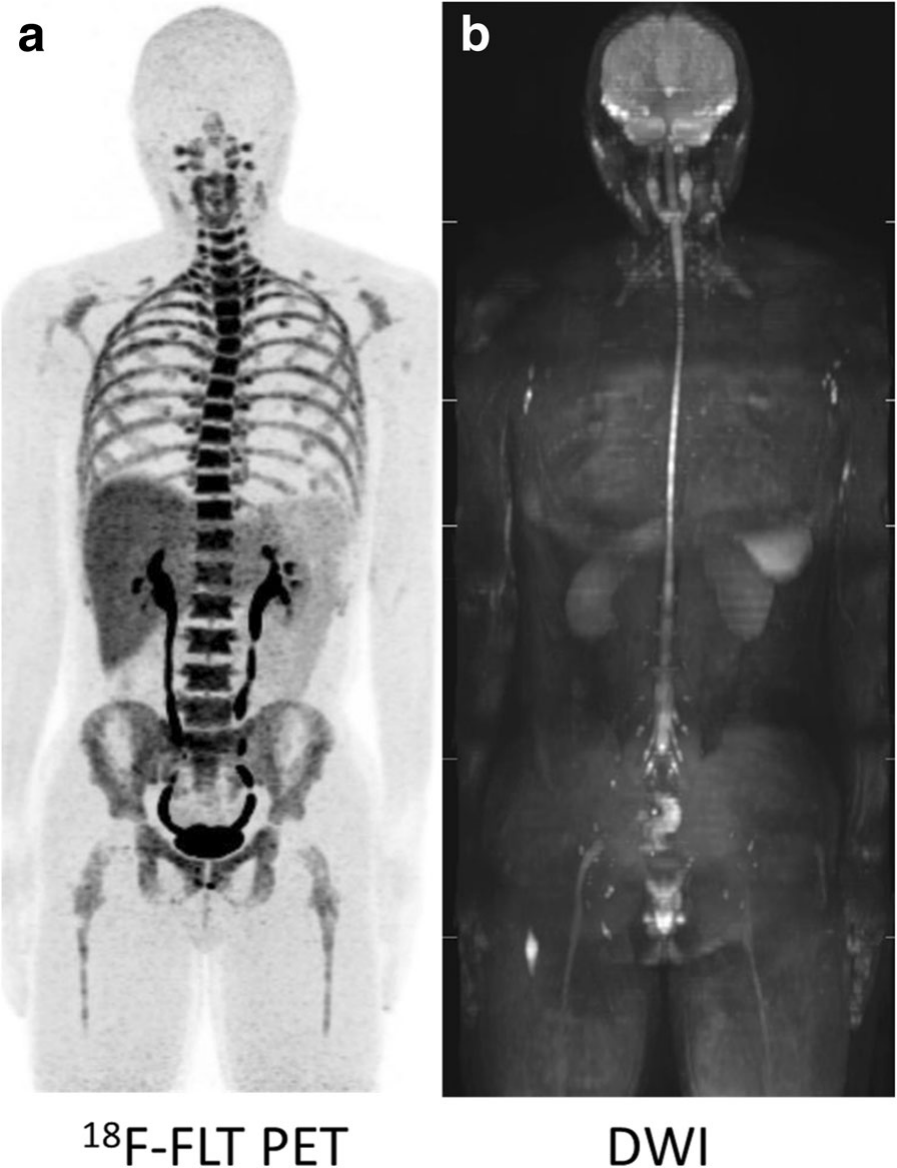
Maximum intensity projection ^18^F-FLT PET (a) and DWI (b) images of a healthy subject. ^18^F-FLT PET clearly visualizes whole-body hematopoietic activity consistent with red bone marrow distribution (a). Whole-body DWI visualizes some organs, such as the spleen, kidneys, spinal cord, and lymph nodes, whereas red bone marrow is invisible or partially visible in normal subjects (b)
